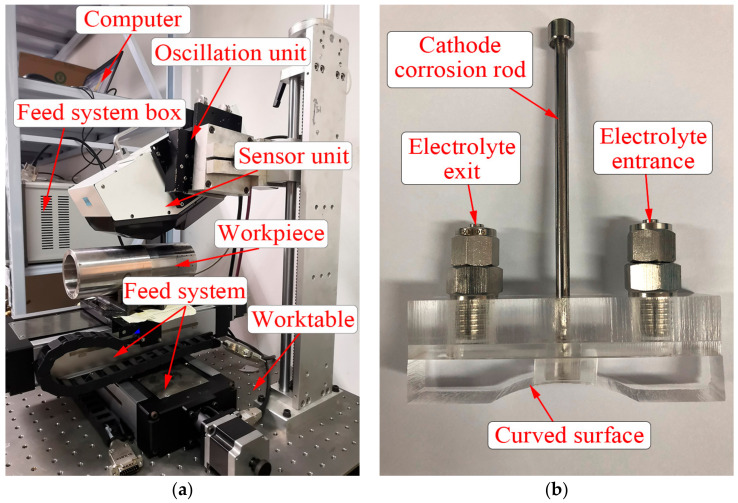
(a) The details of X-ray residual stress measurement; (b) The electrolytic corrosion device.
